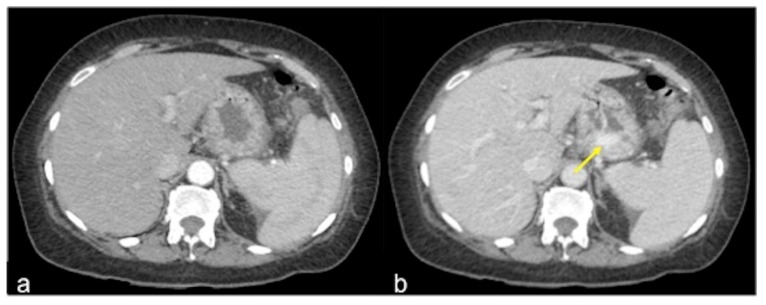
CTA multiphasic study of venous gastric bleeding in arterial (a) and venous (b) phases. In the venous phase, it is possible to see an amorphous haemorrhagic collection ((b) arrow), not detectable in the arterial phase.
